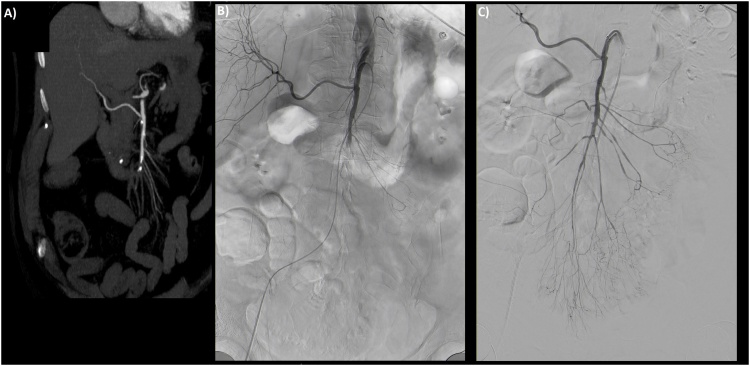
Radiologic imaging of the superior mesenteric artery (SMA) occlusion and subsequent endovascular thrombectomy. a) Contrast enhanced CT angiography with distal SMA occlusion, b) Angiography of the SMA before thrombectomy with perfusion of the proximal jejunal branches and an aberrant origin of the right hepatic artery. c) Successful recanalization of the SMA and its branches.
